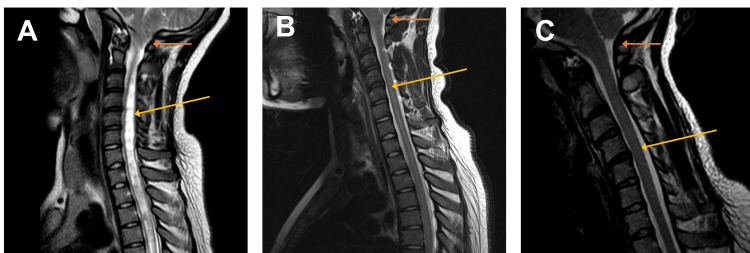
T2-weighted MRI of the cervical spine (sagittal section) (A) Large syringomyelia with extension into the thoracic spinal cord (yellow arrow) and tonsillar herniation (orange arrow) in keeping with Chiari 1 malformation. (B) Improvement of the syrinx (yellow arrow) and improvement of tonsillar descent (orange) at eight months post treatment for thyrotoxicosis. (C) Resolution of the syrinx (yellow arrow) and tonsillar descent (orange) at 20 months post treatment for thyrotoxicosis.
